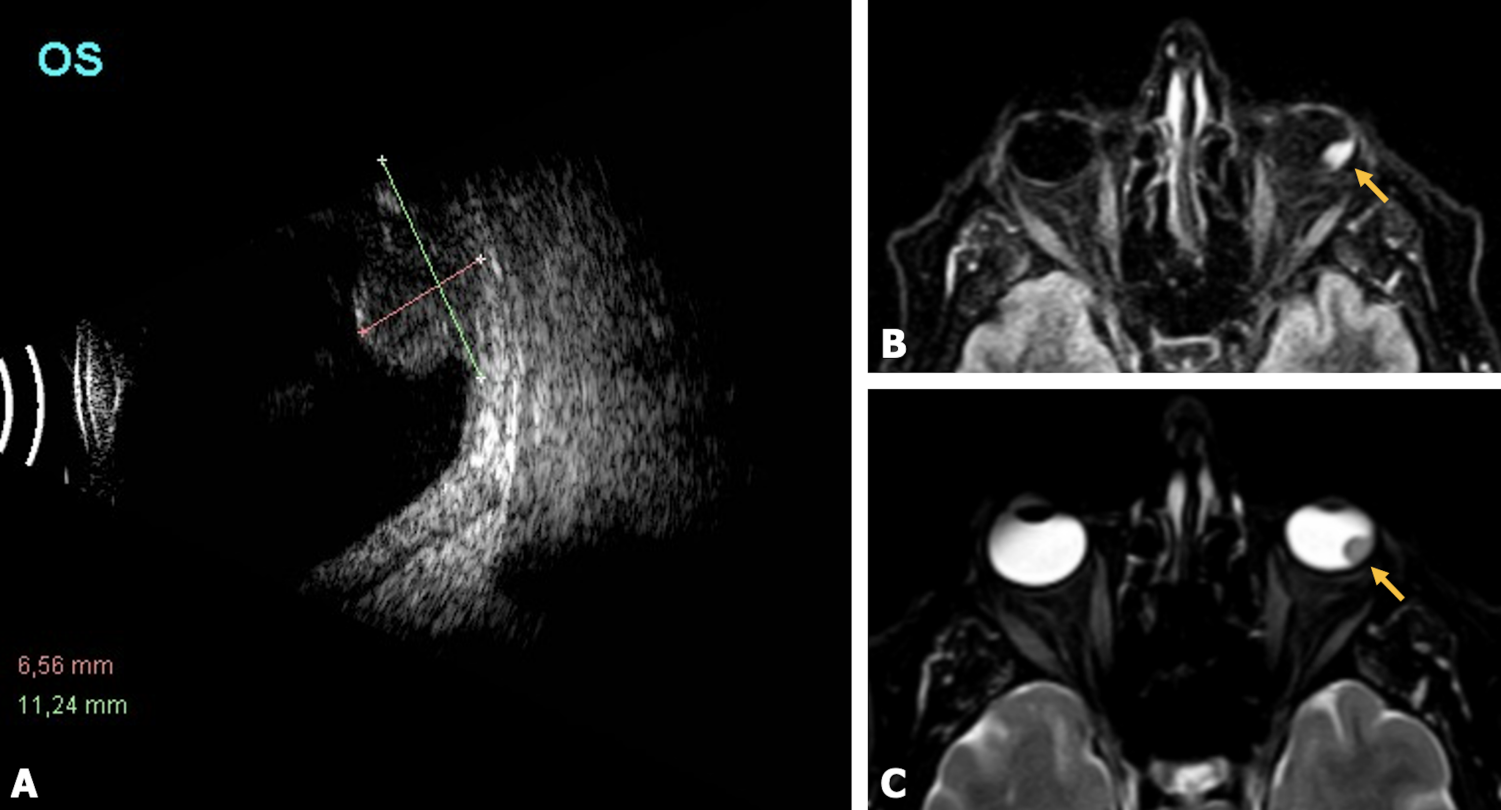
A: B-scan ultrasonography showing a mushroom-shaped mass protruding through the retina into the vitreous cavity, measuring 11.24 mm in the largest basal diameter and 6.56 mm in height. B: Sequence T1 and C: sequence T2 magnetic resonance imaging (MRI) showing a hyperintense and hypointense intraocular mass (yellow arrowhead), respectively, consistent with choroidal melanoma.
